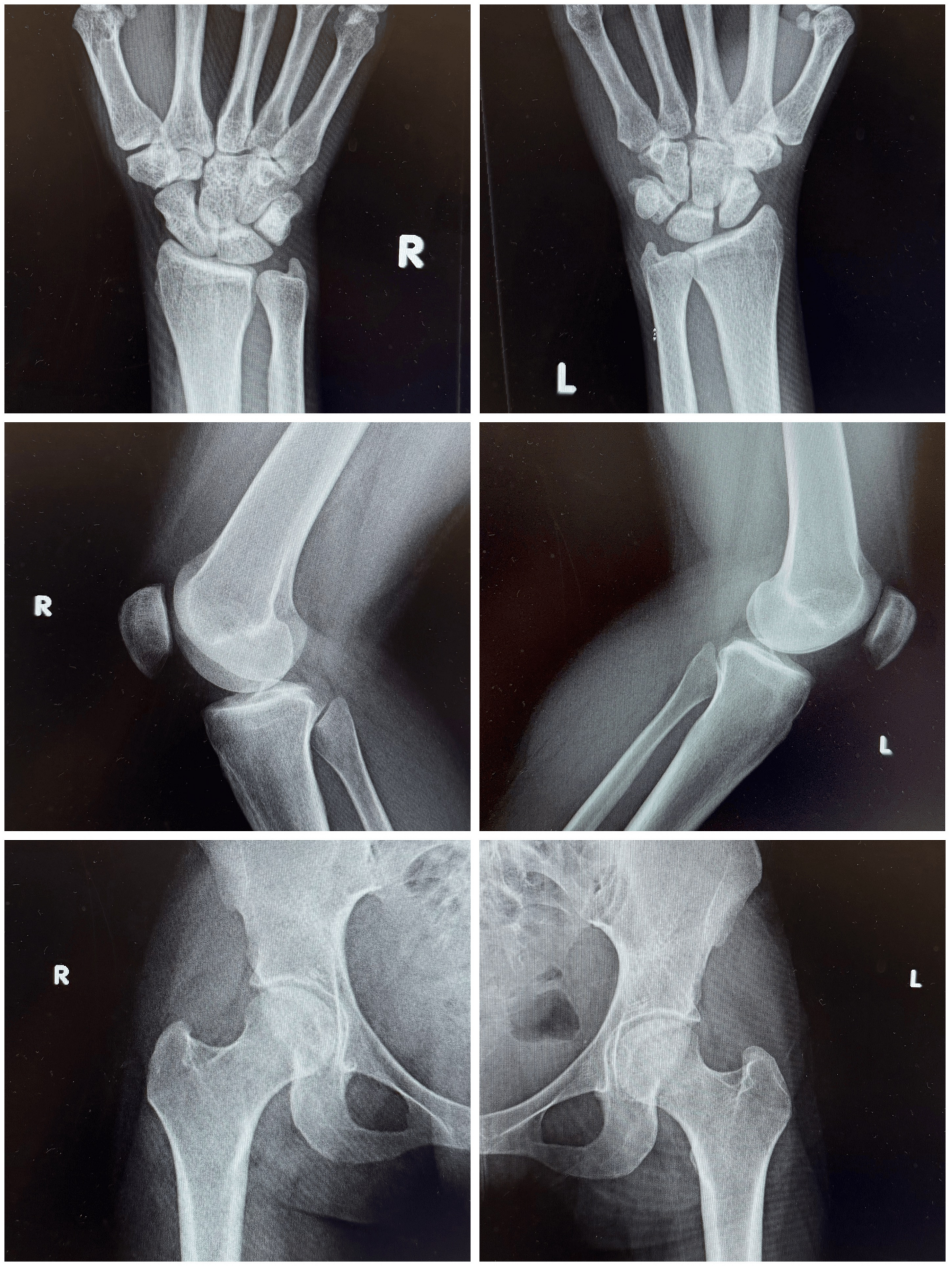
Radiographs of the bilateral wrists, knees, and joints on the day of admission, showing clear joint spaces and well-defined bone structures. There is no evidence of joint space narrowing, effusions, or osteopenia, supporting a noninfectious etiology of the joint pain.
